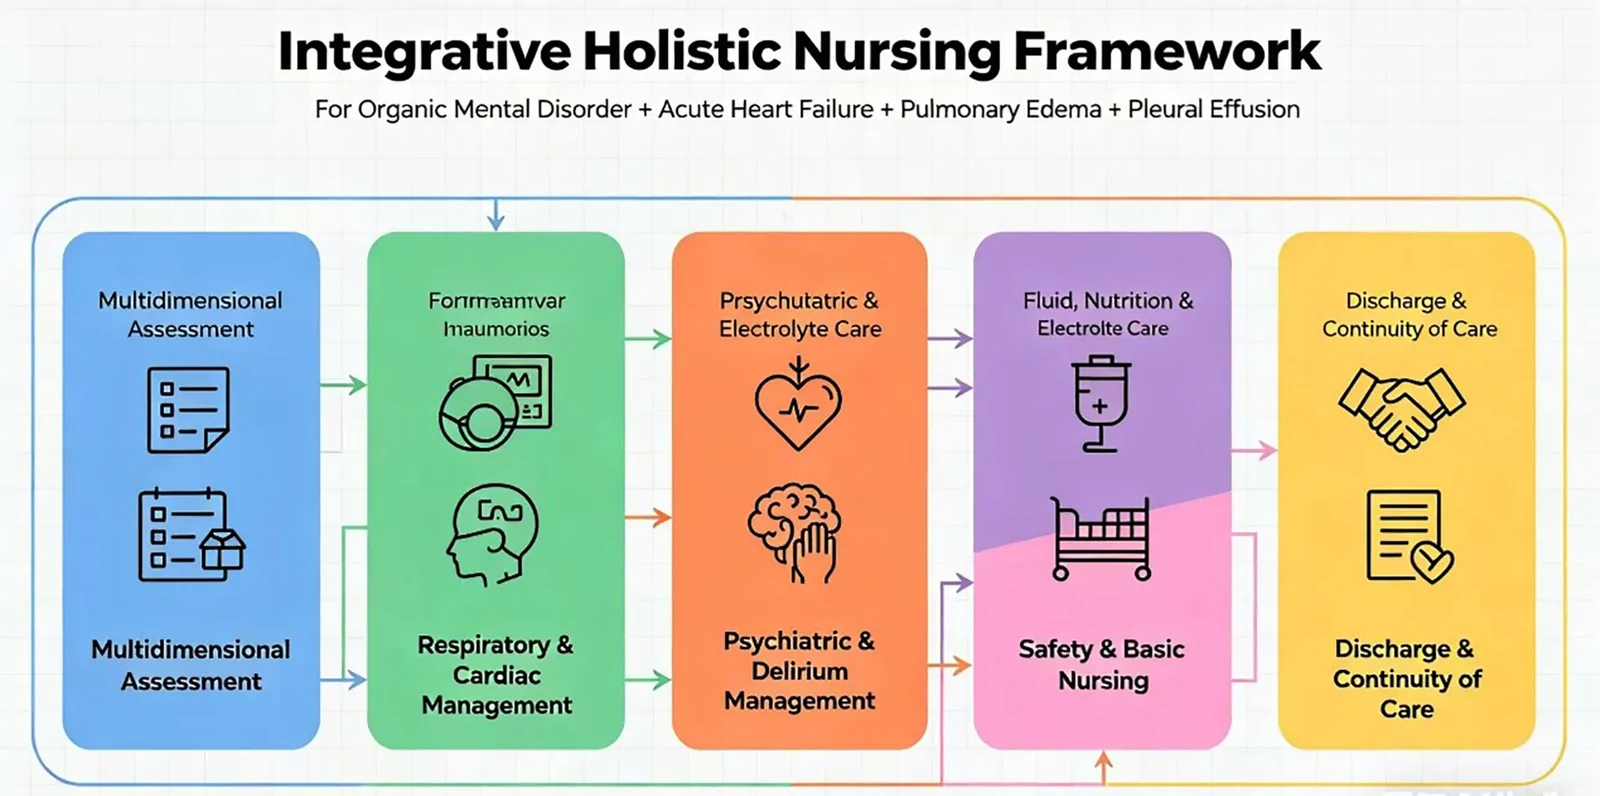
Integrative holistic nursing framework for a patient with organic mental disorder complicated byAHF, pulmonary edema, and pleural effusion. The framework comprises 5 sequential yet interrelated modules: multidimensional assessment (consciousness, respiration, circulation); respiratory & cardiac management (high-flow oxygen therapy, positional management, ultrasound-guided fluid adjustment); psychiatric & delirium management (daytime cognitive orientation, nighttime light sedation); fluid, nutrition & electrolyte care and safety & basic nursing (precise volume management, bundled infection control); and discharge & continuity of care. Arrows indicate the dynamic, bidirectional relationships between modules. This figure was created by the authors based on holistic nursing theory. AHF = acute heart failure.
